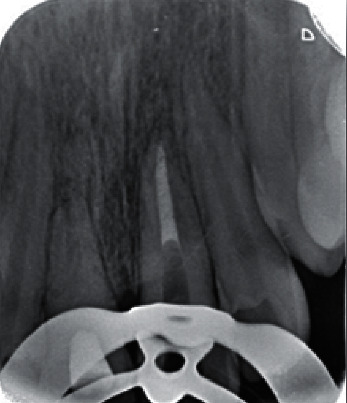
Periapical radiograph taken after one-session apexification of tooth #9 with MTA plug.
